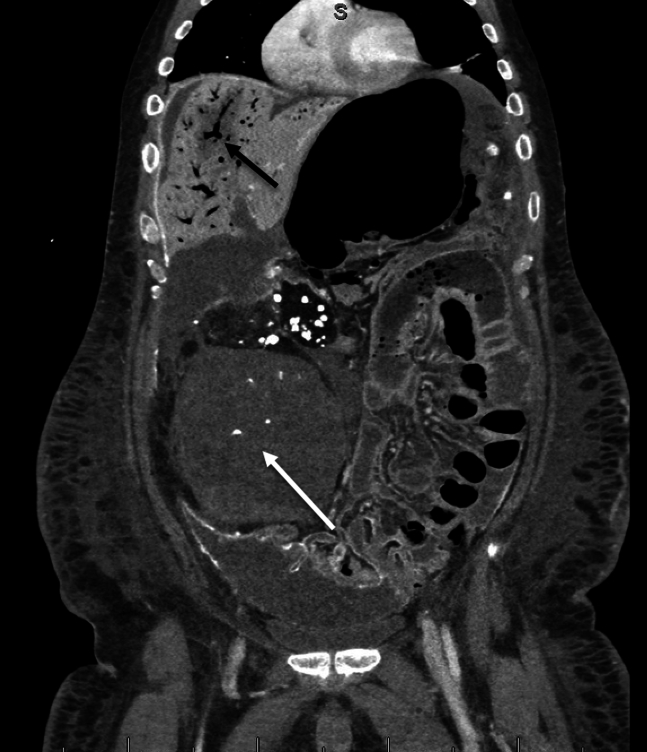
Extensive portal venous gas (black arrow) and small bowel pneumatosis on CTAP. Known retroperitoneal sarcoma is evident as well (white arrow). CTAP, computed tomography abdomen and pelvis.
